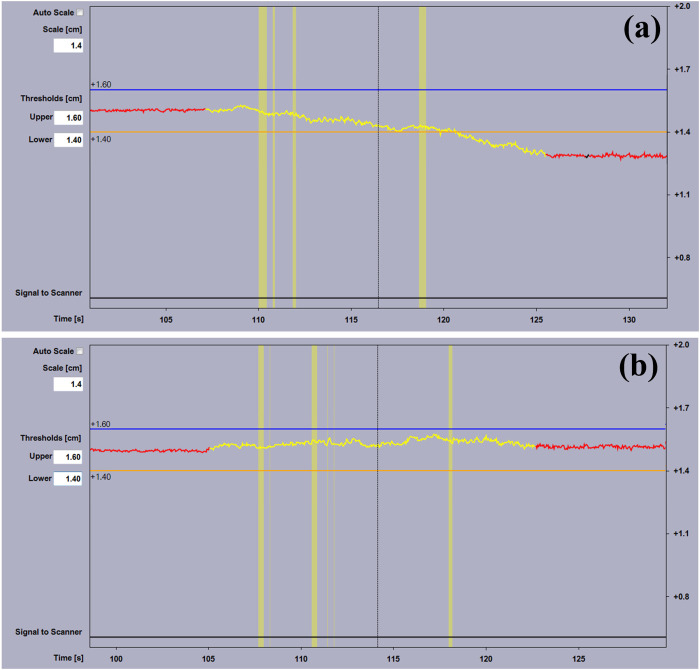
Comparison of different calibration methods. (a) Varian calibration method; (b) The proposed calibration method. The yellow segment indicates the CT beam-on time.
